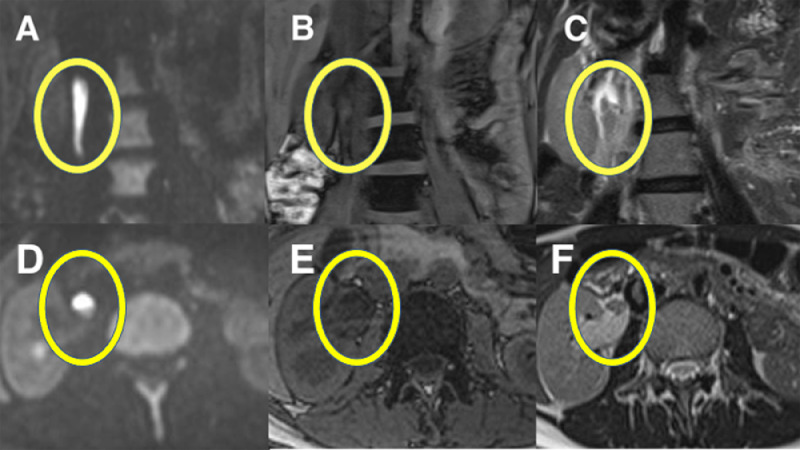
Magnetic resonance imaging shows hydronephrosis of the right kidney and a 4 cm lesion in the renal pelvic-ureteral junction (yellow oval). (A, D) Diffusion-weighted images show high signal intensity, (B, E) T1-weighted images show low signal (with some slightly high-signal intensity) intensity, and (C, F) T2-weighted images show low signal intensity.
